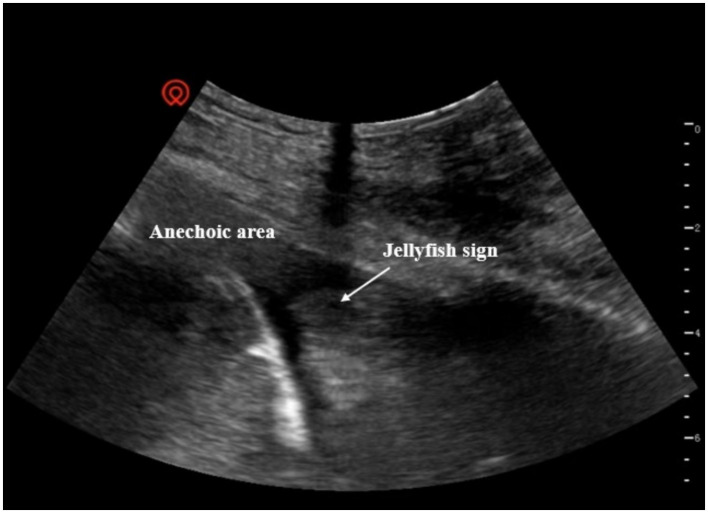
Pleural effusion on ultrasonography. Presence of anechoic areas in the pleural space, accompanied by signs of lung tissue compression.
